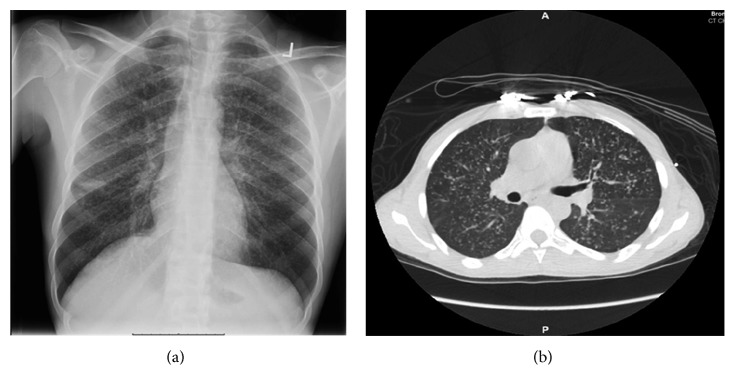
(a) Chest radiograph showing diffuse micronodules. (b) Axial view of the CT chest scan showing diffuse micronodules in a miliary pattern.
